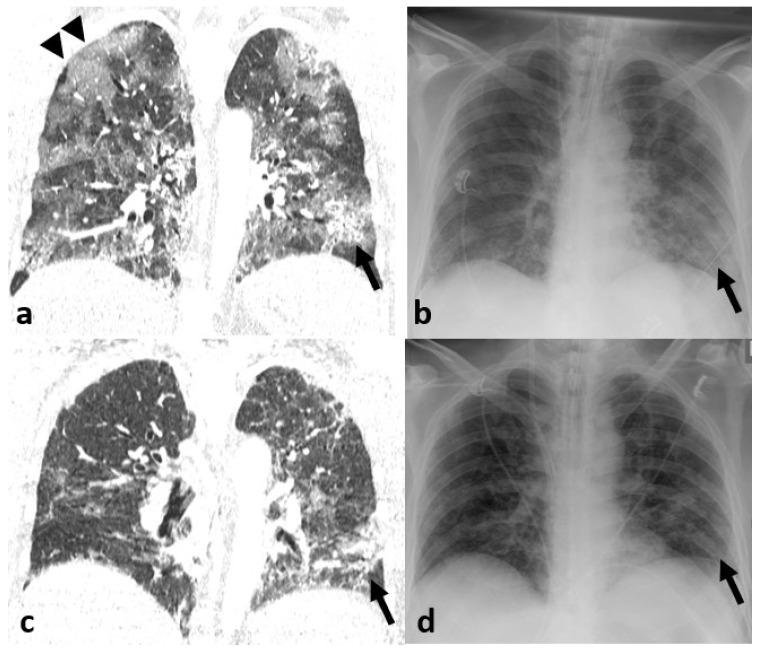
51-year-old male COVID-19 patient with baseline imaging (a,b) and follow-up imaging 21 days after (c,d). At baseline, diffuse ground-glass opacification (GGO) and consolidation in both lobes were present. While consolidation (arrow) is well appreciated on (a) computed tomography (CT) as well as on (b) conventional radiography (CXR), GGOs (arrowheads) were only visible on CT (arrowheads). In the follow-up image, regredient consolidation (arrow) in the left lower lobe can be appreciated on both (c) CT as well as (d) CXR, while changes in the extent of GGO were only appreciated on CT.
